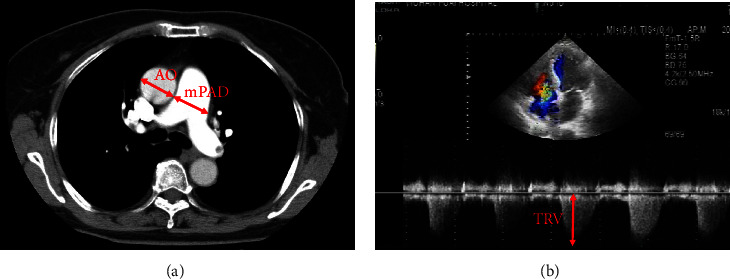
Pulmonary hypertension images by CTPA and echocardiography. (a) mPAD and AO in CTPA (red arrow). (b) TRV was measured (red arrow).
